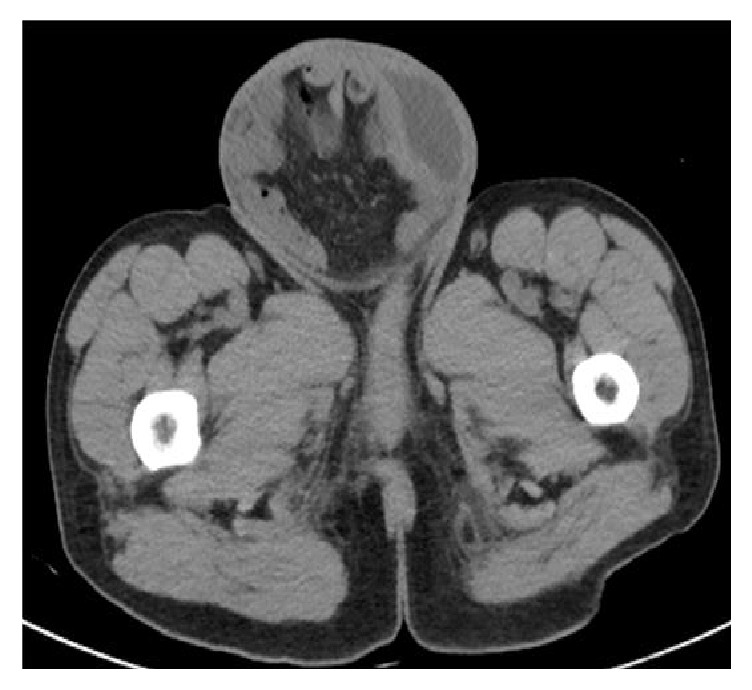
A more inferior CT image demonstrates a portion of the bladder within the very large right inguinal hernia.
